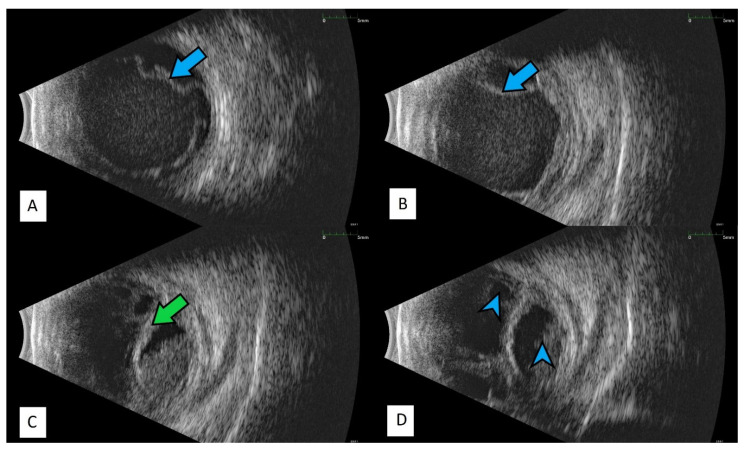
Ultrasound B scans showing the evolution of changes during the development of inflammation. (A,B)—vitritis, posterior vitreous detachment (blue arrow), and retinal thickening. (C,D)—retinal detachment (green arrow) and numerous hyperechoic densities in the vitreous chamber (blue arrowhead).
